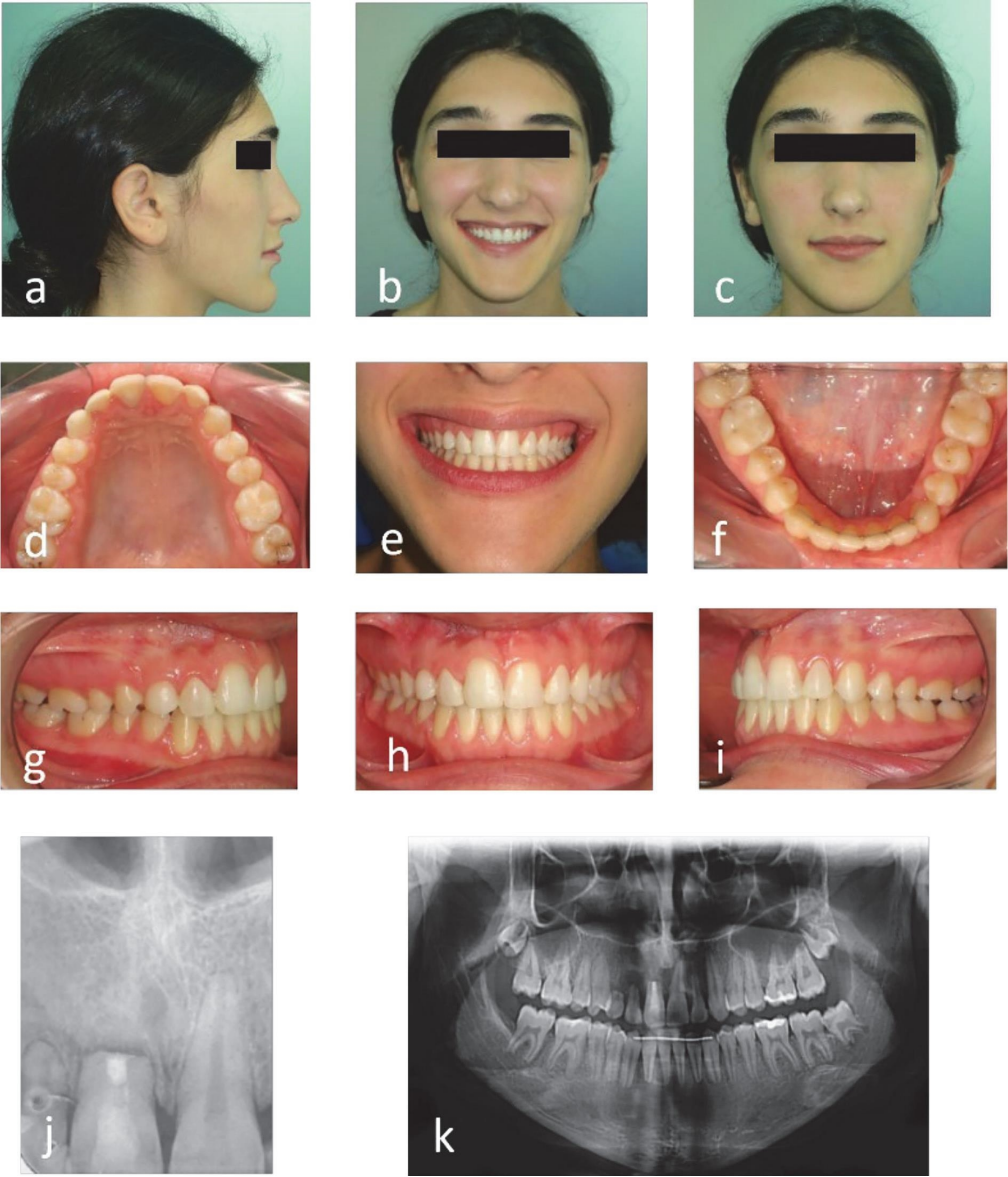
Follow‐up photographs and radiographs 3 years after endodontic microsurgery: (a–c) extraoral view with improved smile esthetics; (d–i) intraoral view with aligned teeth and Class I occlusion; (j) periapical radiograph showing stability of the maxillary right incisor; and (k) panoramic radiograph showing root parallelism and overall dentition.
